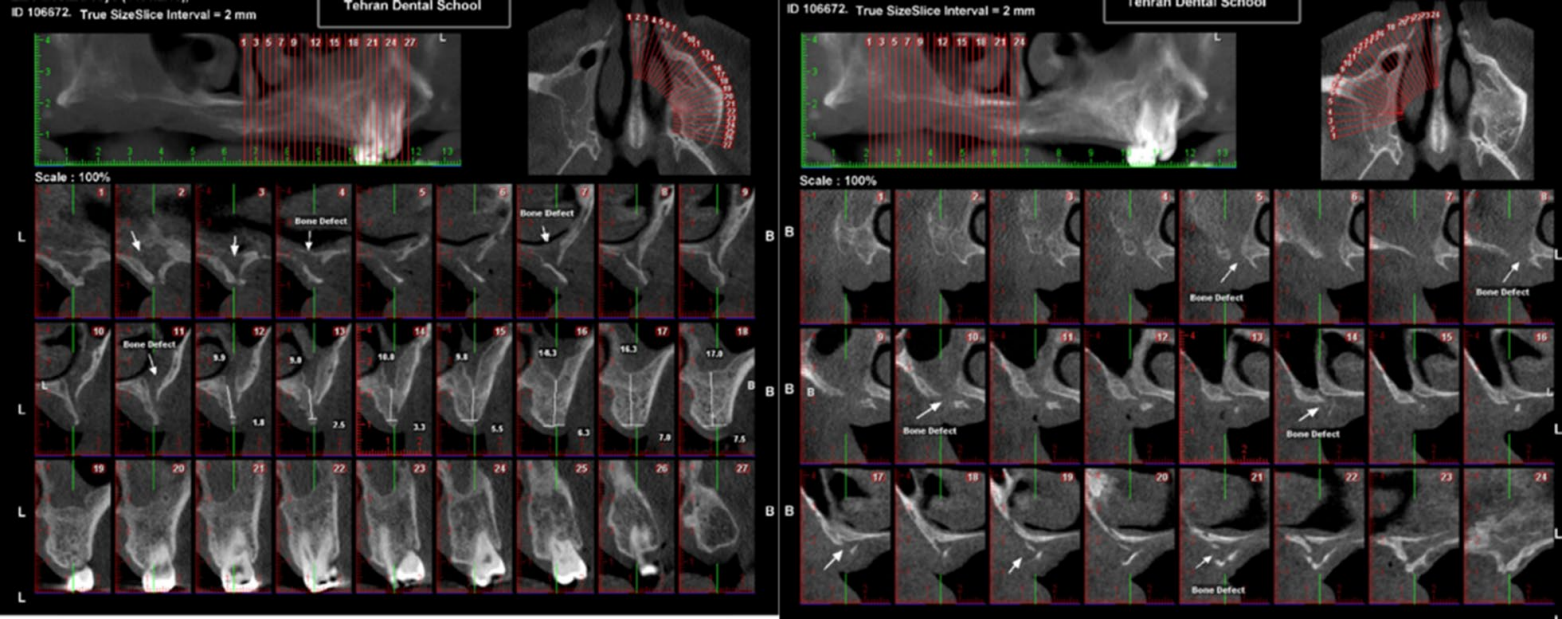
Cone-beam computed tomography after subtotal maxillectomy
